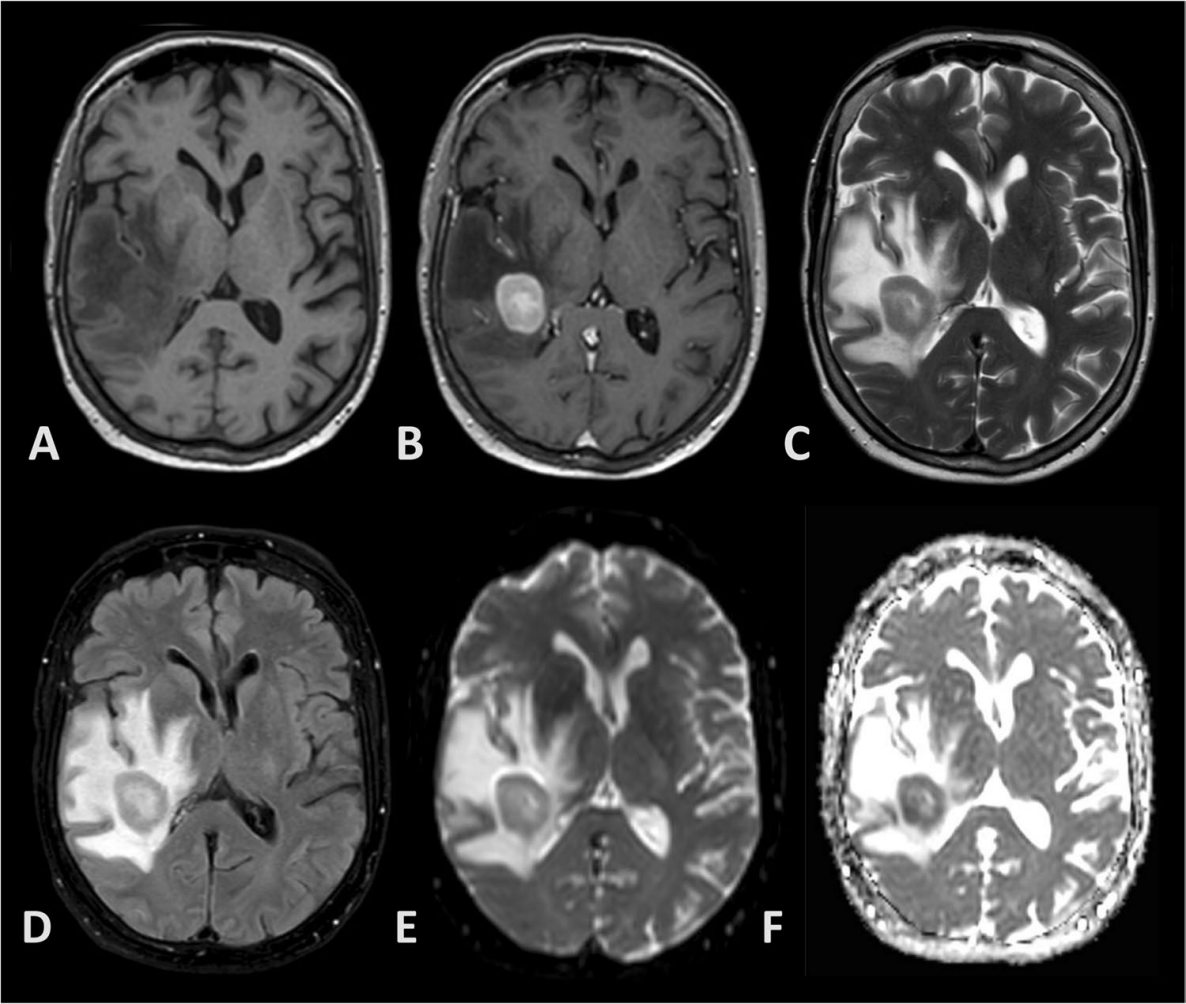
Example of magnetic resonance imaging (MRI) of the brain for patient suffering from primary central nervous system lymphoma (PCNSL). a T1-weighted; b T1-weighted post gadolinium; c T2-weighted; d Fluid-attenuated inversion recovery (FLAIR); e Diffusion-weighted imaging (DWI); f Apparent diffusion coefficient (ADC)
